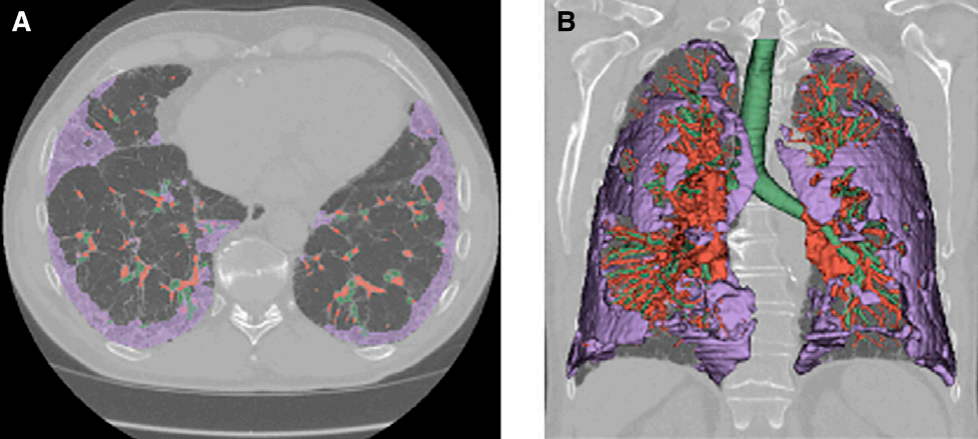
(*A* and *B*) Axial (*A*) and three-dimensional rendering (*B*) of an example high-resolution computed tomography scan that has been segmented to show areas of fibrosis (purple), blood vessels (red), and airways (green) within a lung mask.
